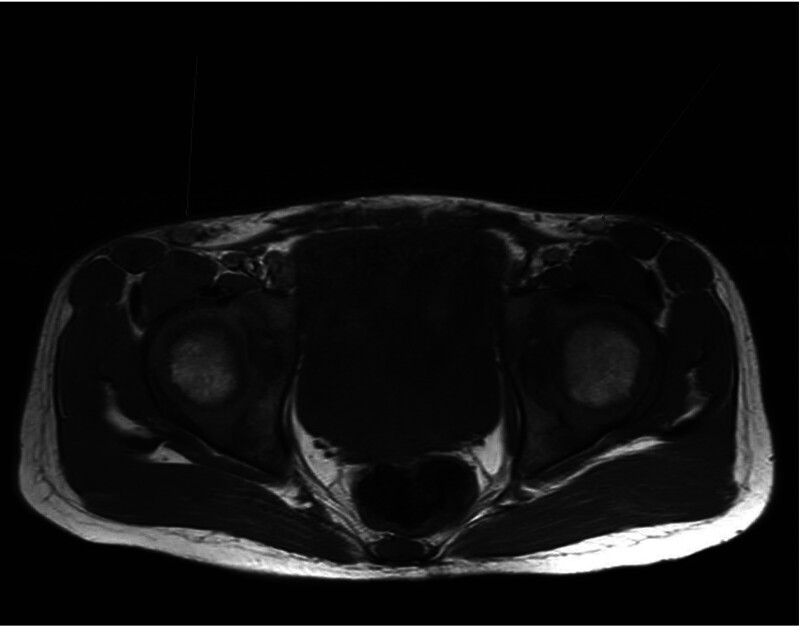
Axial T1-weighted MRI of the pelvis without contrast showing multiple benign-appearing lymph nodes (arrows) in the bilateral inguinal regions. No evidence of pathological lymphadenopathy or osseous lesions was noted. The imaging was performed as part of the preoperative assessment for lower limb lymphedema and protein-losing enteropathy.
